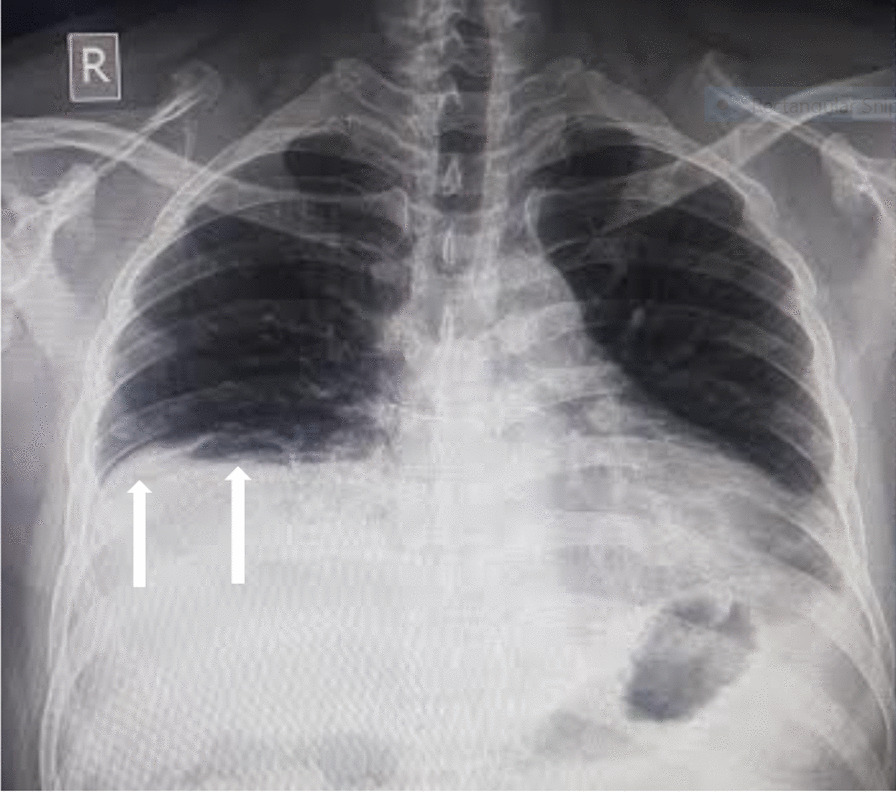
Chest X-ray showing higher level of right hemidiaphragm in comparison to left side (pointed by white arrows). There is also blunting of bilateral costophrenic angles
